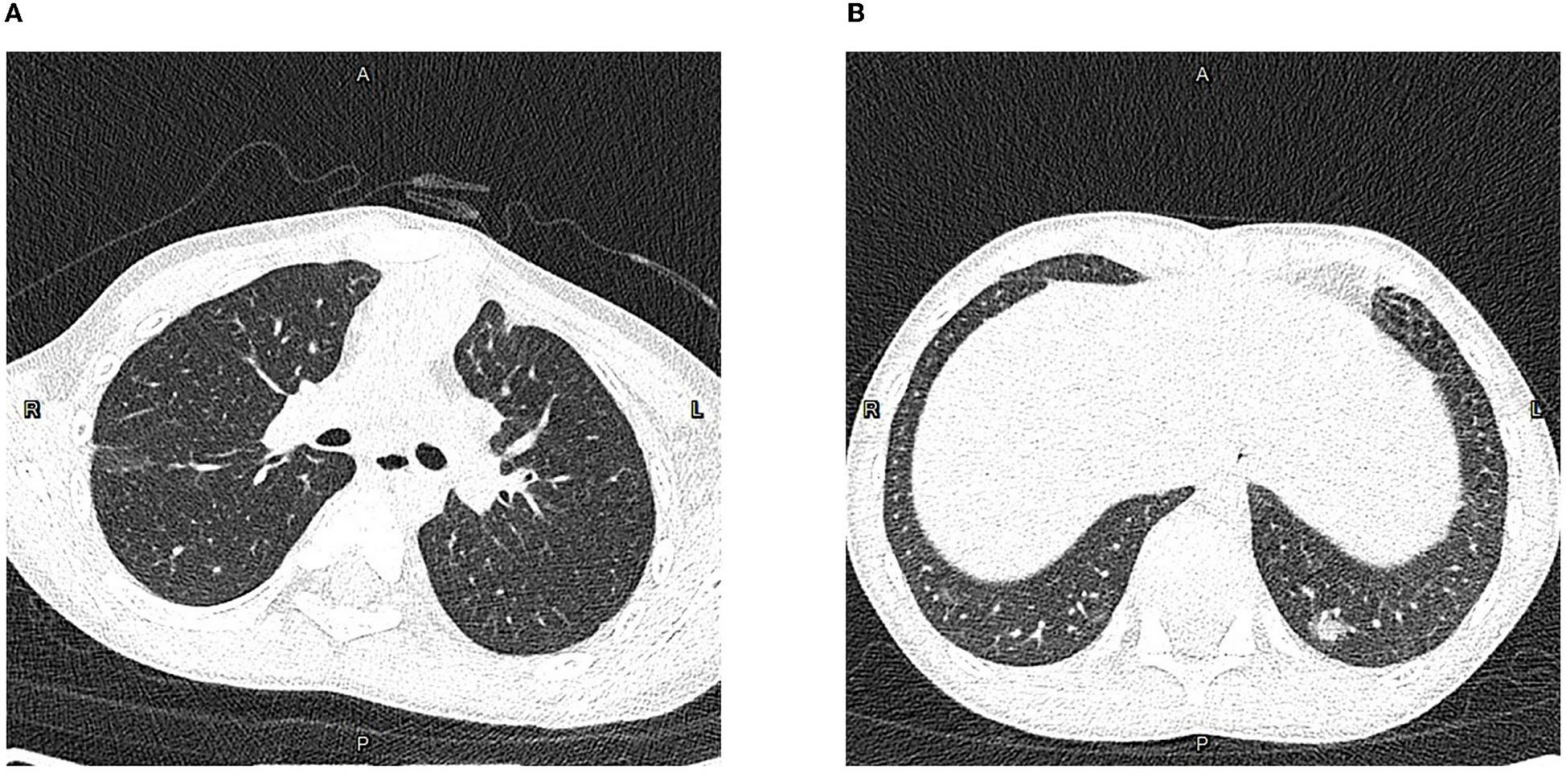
Computed tomography of the chest in the axial plane. (A) On hospital Day (HD) 1, showing slight inflammation in the middle lobe of the right lung and upper lobe of the left lung. (B) On HD 7, showing little emerging flaky exudation in the posterior basal segment of the left lower lobe.
